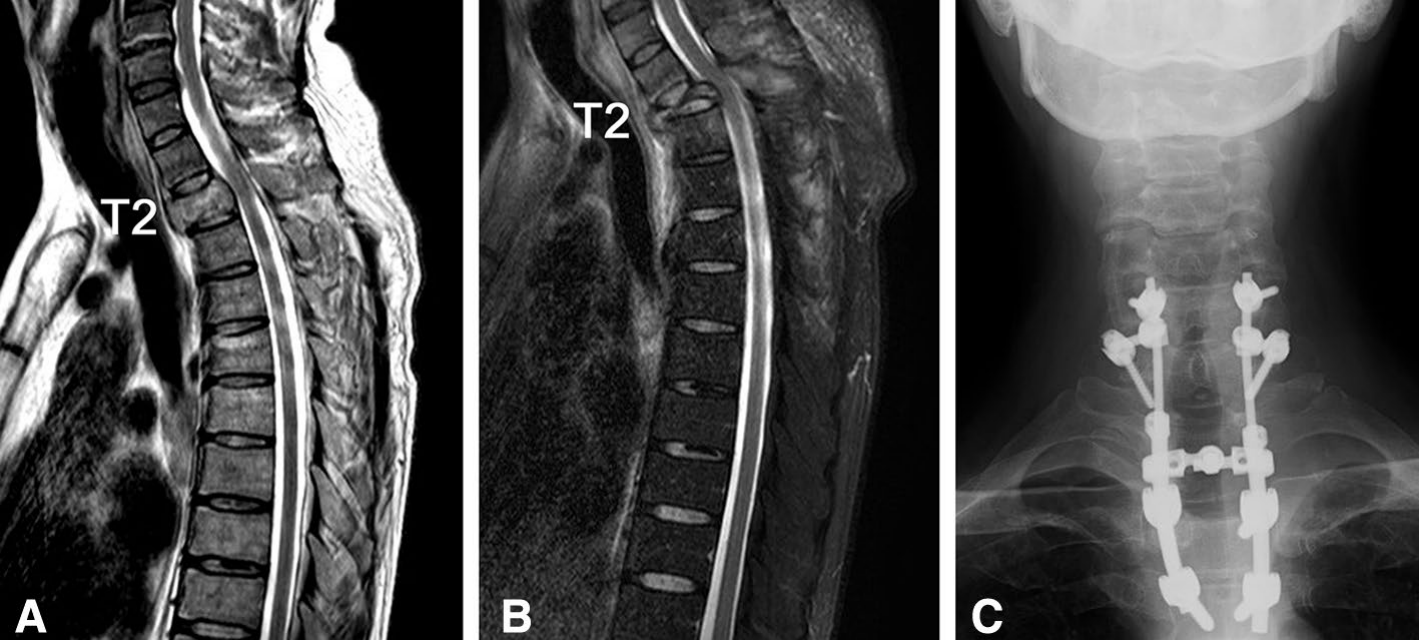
The images of the thoracic column in the patients with MSCC of esophageal cancer. The second thoracic vertebra collapsed, and surgery was performed. a T2-weighted sagittal MRI images before the radiotherapy. b T2-weighted fat suppression sagittal MRI images when the thoracic vertebra collapsed. c Anteroposterior plain radiograph after surgery
